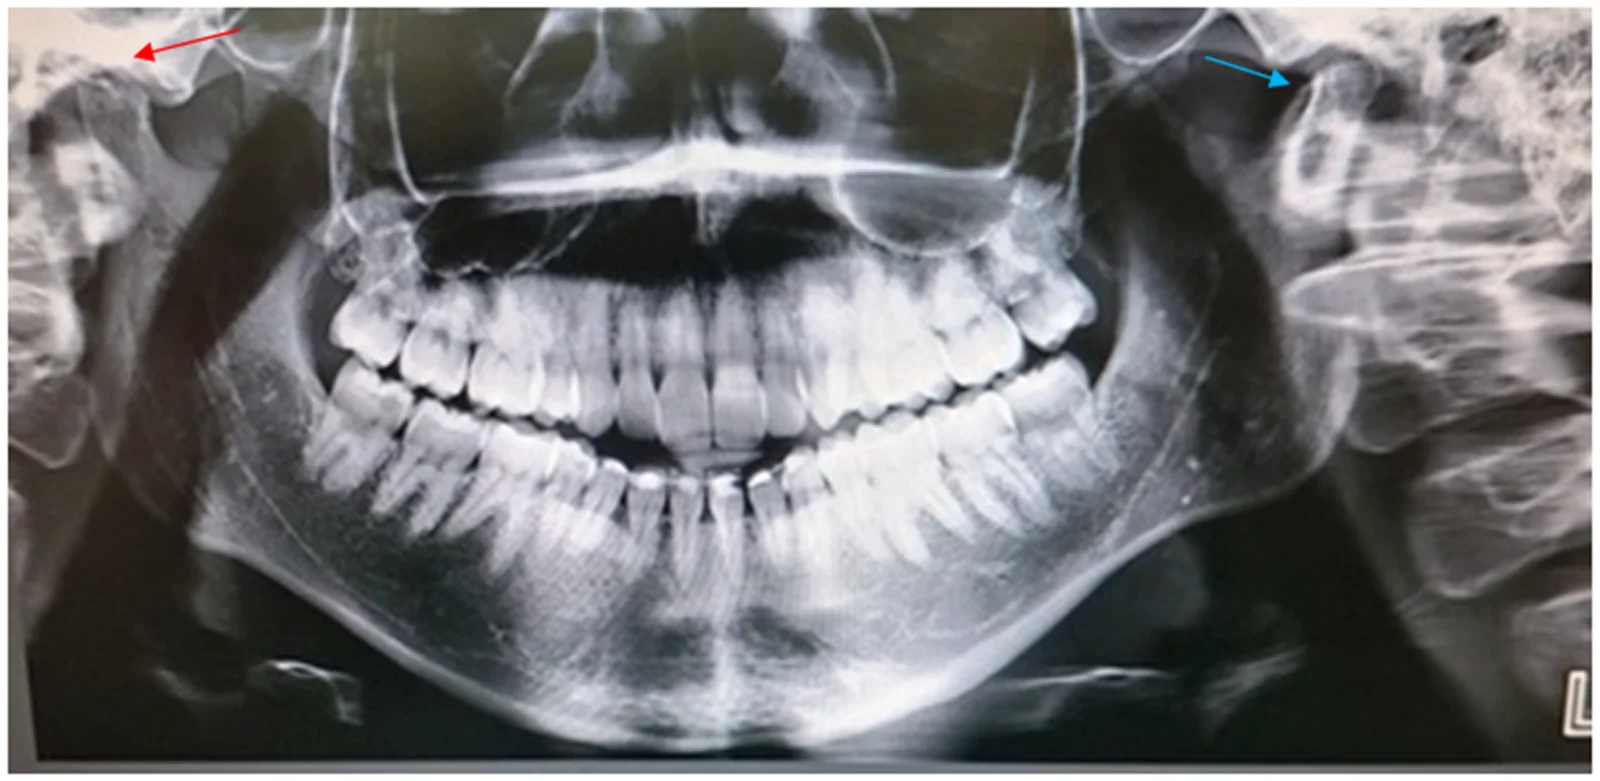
Orthopantomographic imaging of the patient at 27 years of age (a 15-year follow-up) reveals bilateral degenerative alterations of the temporomandibular joints, consistent with the chronic changes in the underlying condition and in agreement with the patient’s clinical findings. The left temporomandibular joint (blue arrow) demonstrates the presence of an intraosseous cyst along with osseous apposition on the condylar head, indicative of osteophyte formation and advanced degenerative remodeling. In contrast, the right temporomandibular joint (red arrow) exhibits flattening of the condylar head accompanied by a reduction in the interarticular space, as a result of the long-standing articular degeneration.
